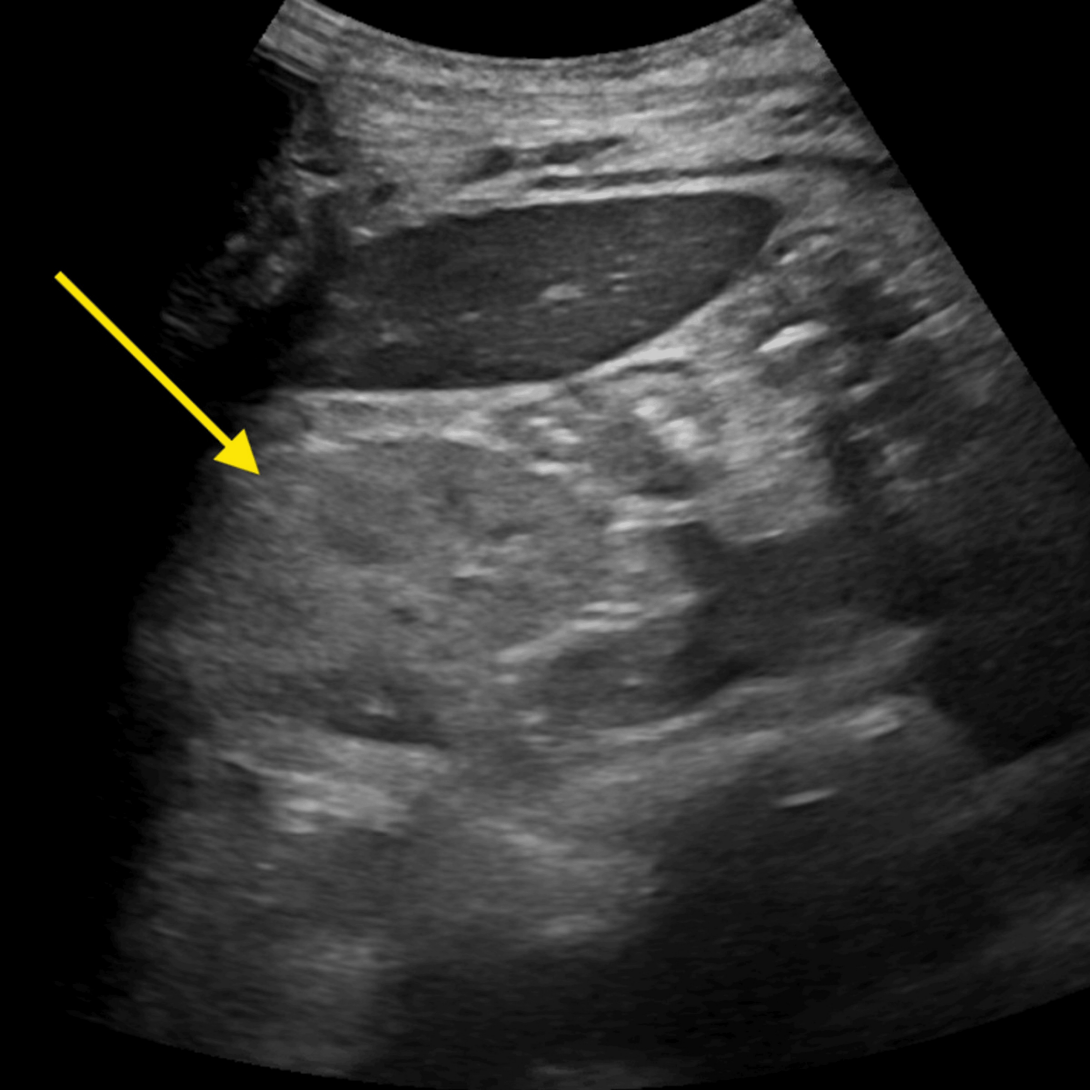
Abdominal ultrasound The abdominal ultrasound revealed a hyperechoic right kidney.
